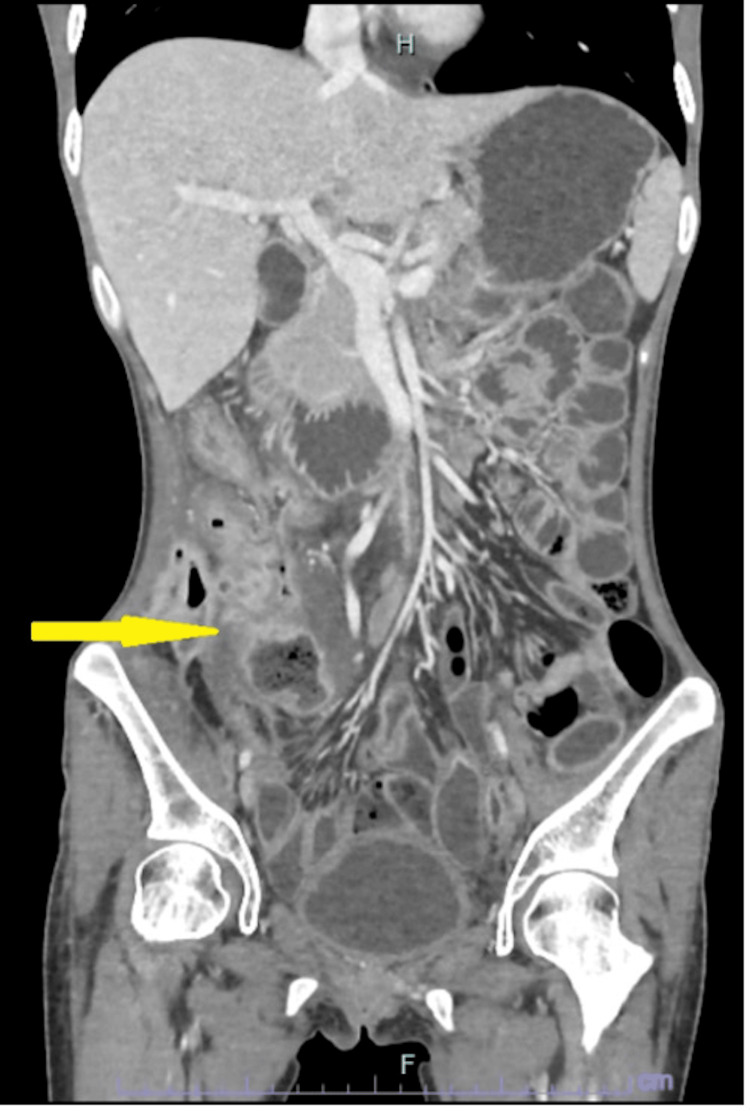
CT scan (coronal view) showing a heterogenous collection (yellow arrow) at the right paracolic gutter with small air locules within.
